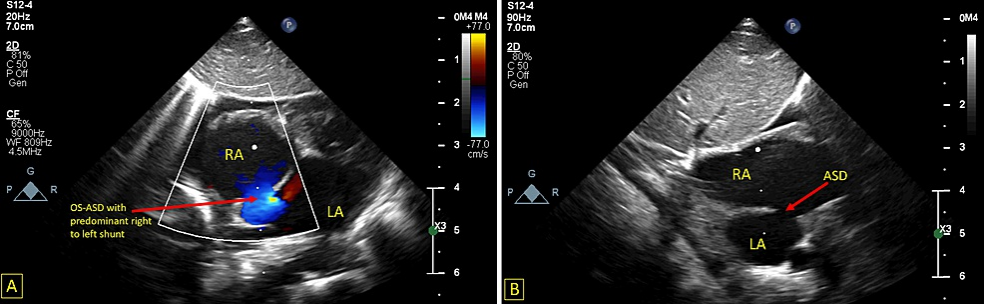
A: Showing a subcostal view with color Doppler demonstrating an ostium secundum atrial septal defect with predominant right to left shunt. B: Subcostal bicaval view showing the atrial septal defect. LA – Left atrium, RA – Right atrium, OS-ASD – Ostium secundum atrial septal defect
